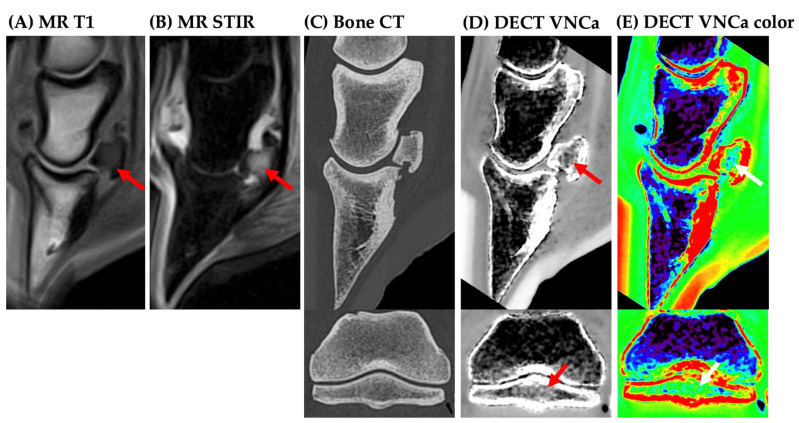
A standing 24-year-old Bradenburger with acute lameness of unknown origin and a severe diffuse bone edema-like lesion (BME) of the navicular bone (arrow). (A) Diffuse T1-hypointensity of the navicular bone; (B) diffuse short-tau inversion recovery sequence hyperintensity of the navicular bone; (C) standard bone CT (sagittal and transverse plane) within normal limits; (D) DECT virtual-non-calcium map (VNCa) map (sagittal and transverse plane)—diffuse hyperdensity of the navicular bone; (E) color DECT VNCa map (sagittal and transverse plane)—BME is displayed in green in contrast to the normal fatty bone marrow in the distal and middle phalanx (blue).
